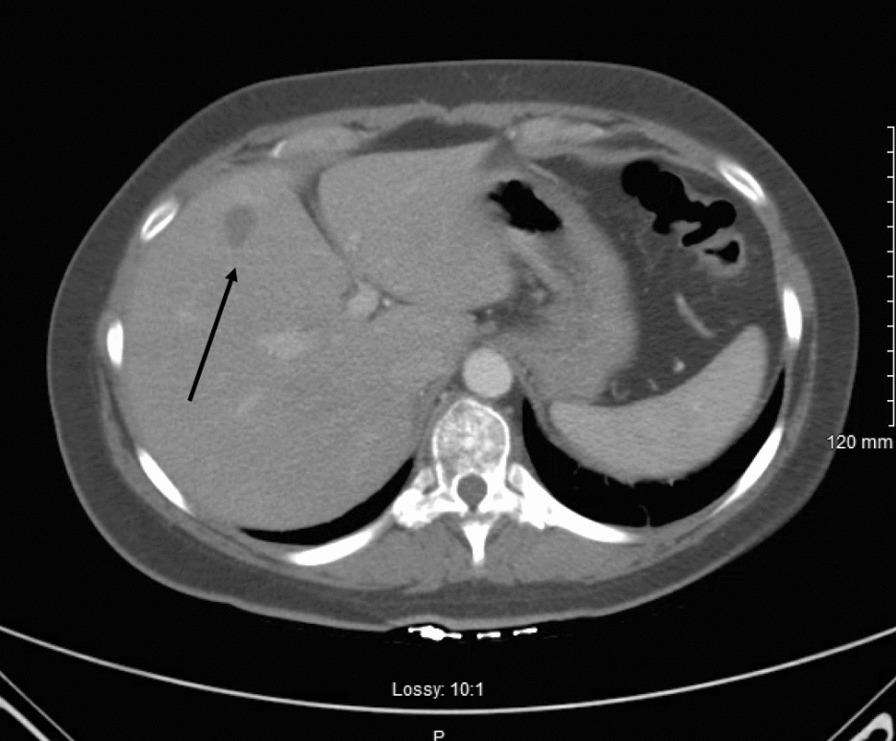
Axial section of a CT of the abdomen and pelvis with intravenous contrast revealing a 1.2-cm mass (arrow) in the right lobe of the liver
